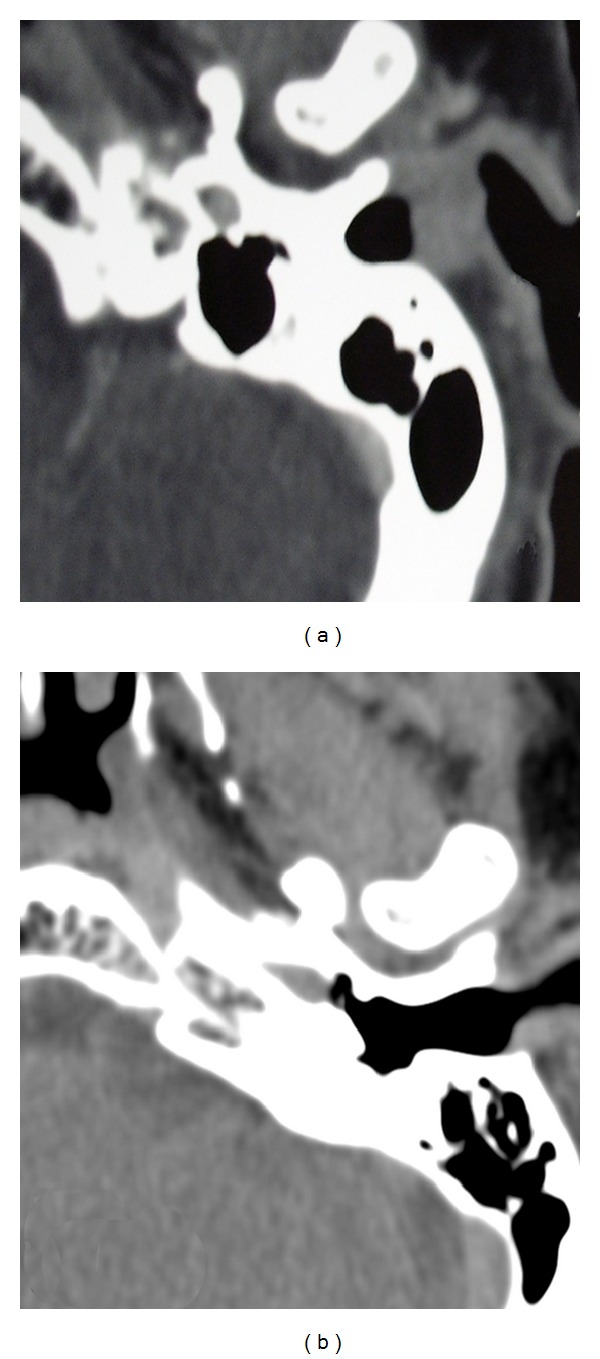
Petrous bone CT before (a) and after (b) the excisional biopsy.
